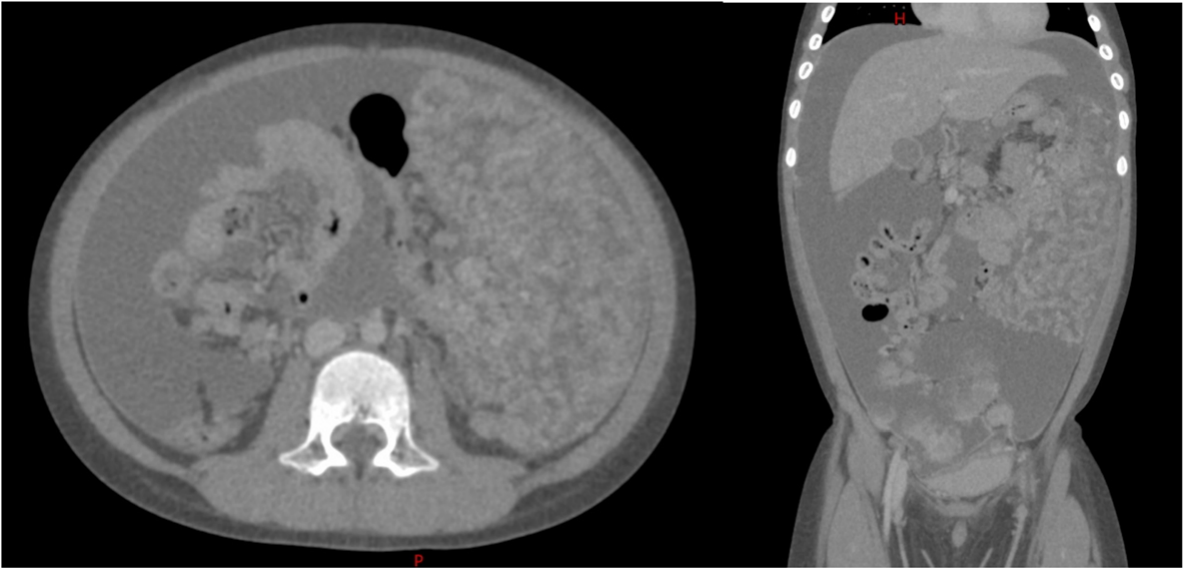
Computed tomography image obtained upon diagnosis showing multiple solid tumors in the pelvis, with a thickened omentum and ascites
